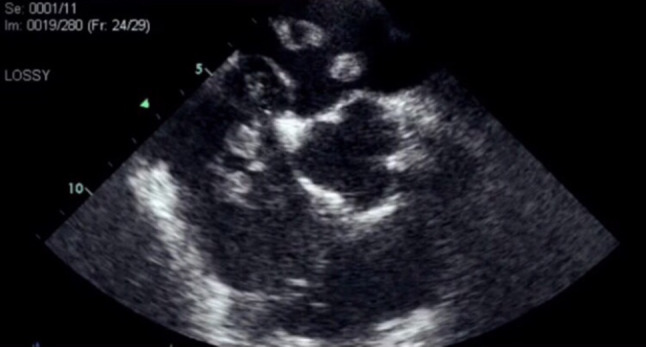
Transoesophageal echocardiogram, short axis view, displaying the masses within both atria
